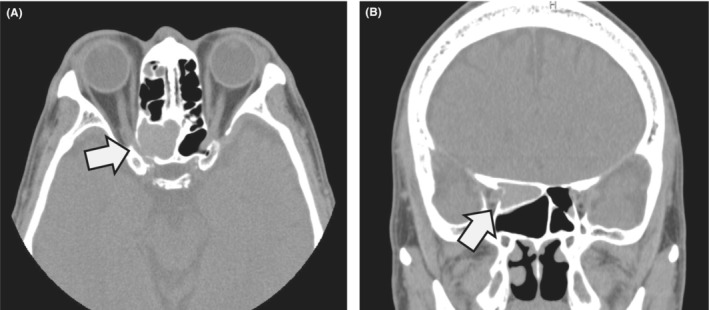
Paranasal cavity CT scan. Mucosal thickening and liquid retention in the right ethmoid sinus with accompanying destruction of the adjacent bone and compression of the optic nerve (A: axial, arrow and B: coronal, arrow).
